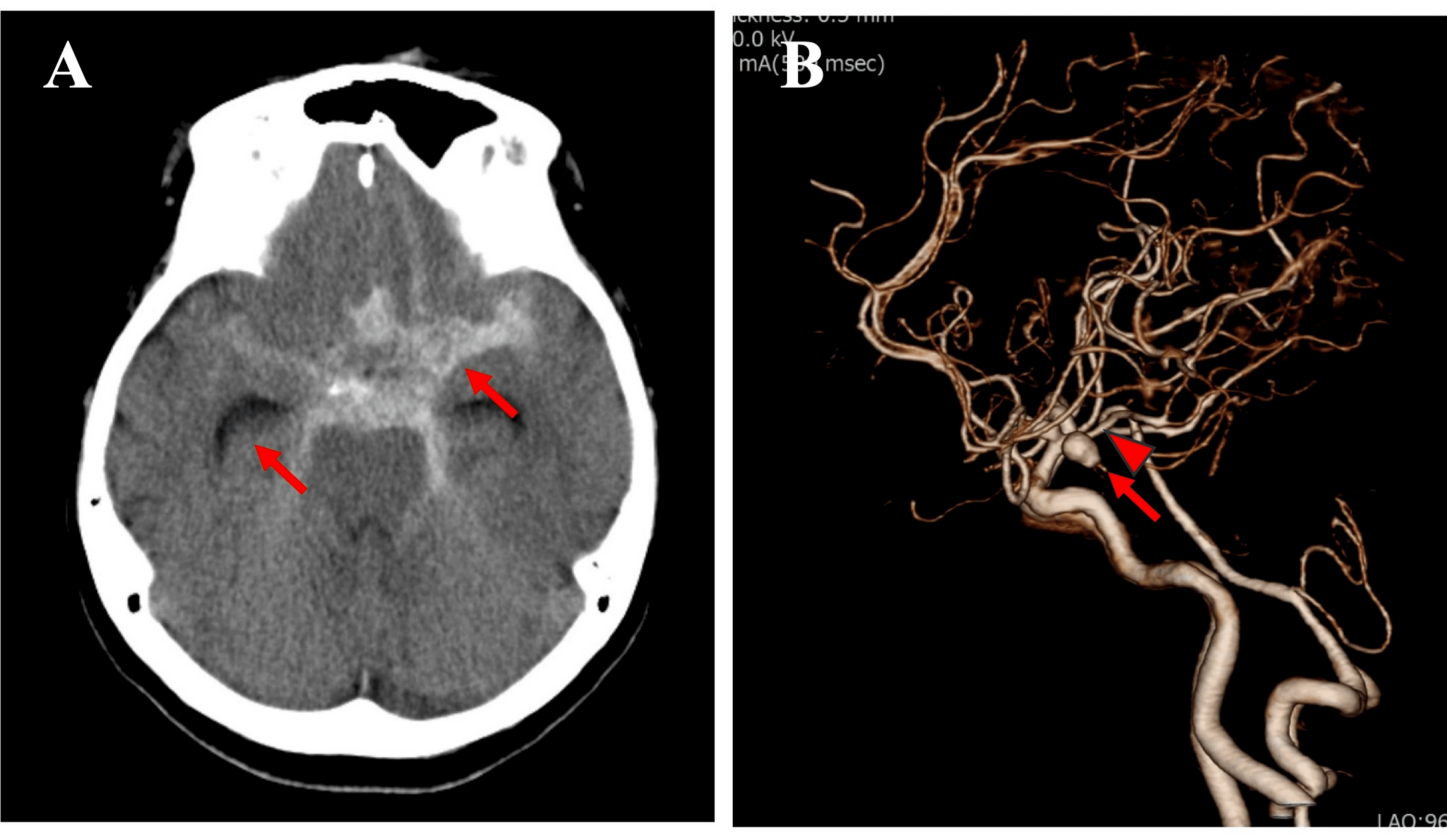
Neuroradiological findings on admission. (A) Non-contrast head computed tomography (CT) showing subarachnoid hemorrhage with ventricular enlargement (red arrows).
